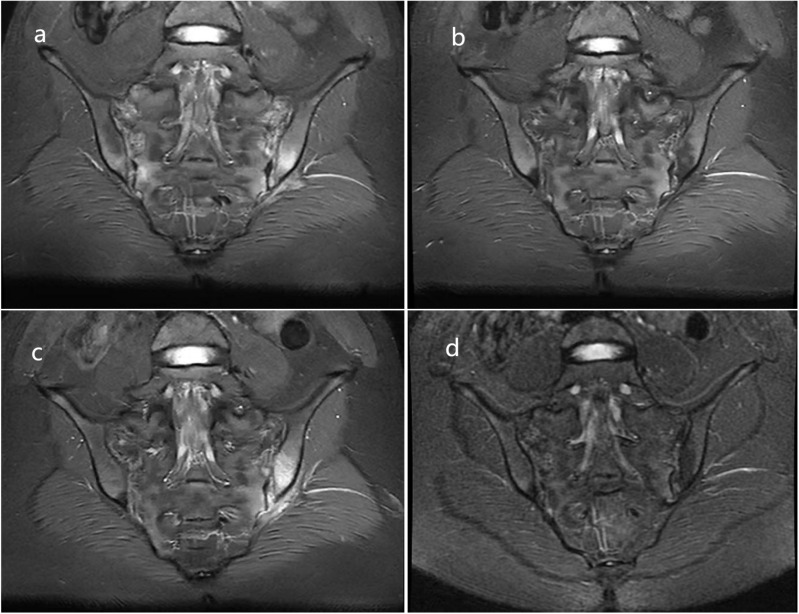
Sacral-iliac joint MRI: Before adalimumab treatment (A) and 6 months after adalimumab treatment (B); Before secukinumab and upadacitinib treatment (C) and 6 months after upadacitinib treatment (D).
